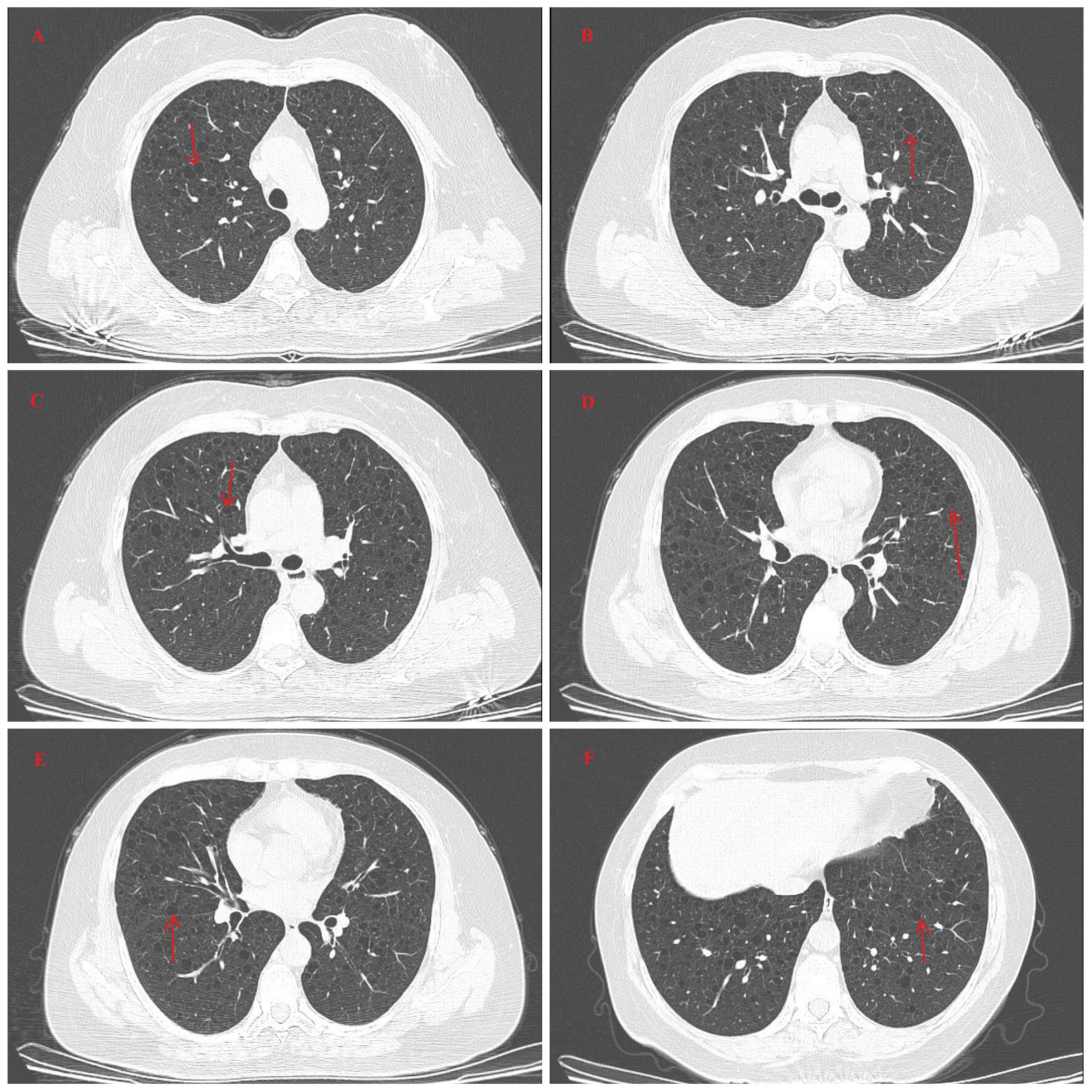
Lung CT images (October 2024) (A–F) HRCT of the lung demonstrates multiple pulmonary bullae (arrows) and diffuse emphysema in both lungs. HRCT: high-resolution computed tomography
